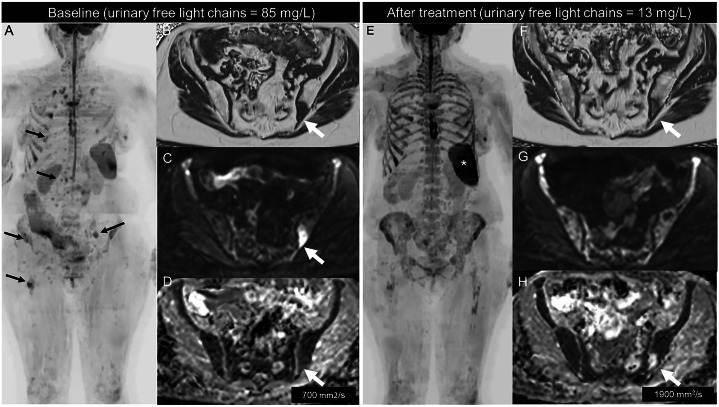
Whole-body MRI with MY-RADS protocol in a patient with relapsing light chain multiple myeloma, before (left) and after (right) treatment with Daratumumab, pomalidomide and dexamethasone and bone marrow stimulating factors. Bone involvement with a focal pattern can be noted in multiple skeletal regions in the coronal maximum intensity projection of high b-value DWI, displayed in inverted grayscale (arrows in A). Axial sections show a focal lesion in the left iliac bone (arrows) with low fat content in the fat fraction map (B), high signal intensity in high b-value DWI (C), and low ADC values (D). After treatment, diffuse intermediate intensity signal is visible on DWI images (E) across the whole skeleton, without corresponding bone marrow infiltration on fat fraction; spleen enlargement is also present (asterisk in E). Notably, this finding is related to the administration of bone marrow-stimulating factors and does not indicate disease progression. On the other hand, focal lesions such as the one in the left iliac bone show decreased signal in DWI and are not visible (E, G), initial fatty repopulation (arrow in F) and markedly increased ADC (arrow in H), findings suggesting high likelihood of response (RAC 1)
